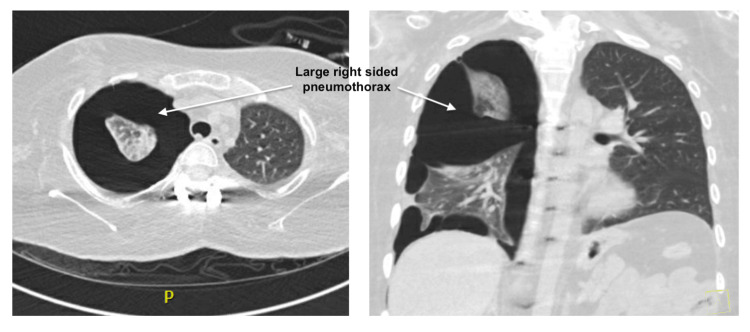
CT of the chest showing a large right-sided pneumothorax with portions of the right lung attached to the lateral chest wall due to adhesions
